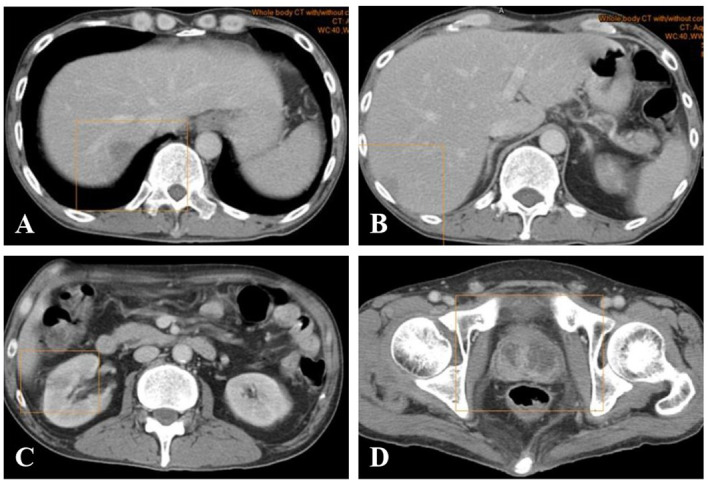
Whole-body computed tomography revealed (A, B) poorly enhanced hypodense lesions in segment VII of the liver, consistent with hepatic abscesses; (C) a 1.5-cm abscess in the right kidney; and (D) a hypodense lesion within the prostate, later confirmed as a prostatic abscess on drainage.
